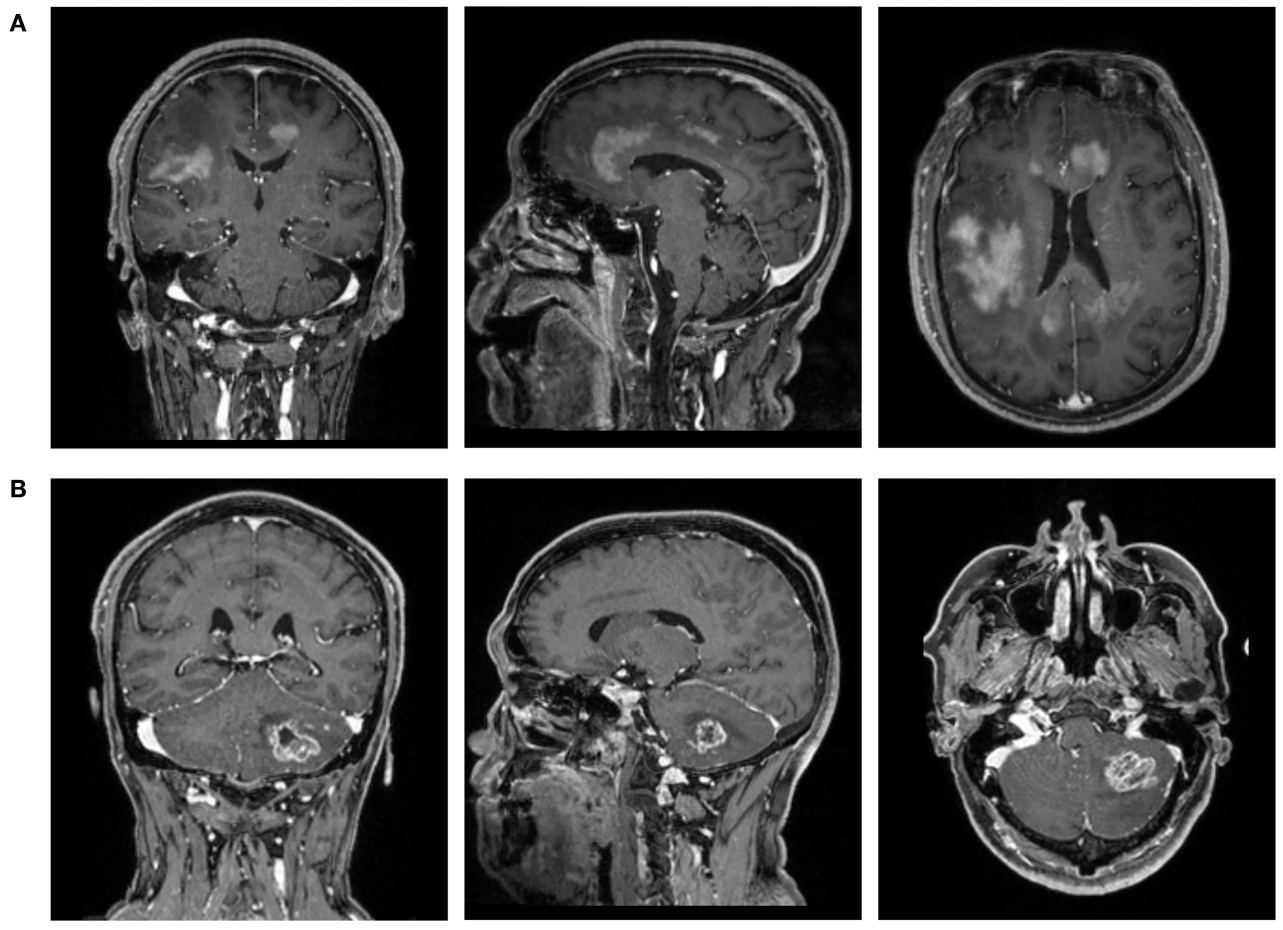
The magnetic resonance images (T1C) of patients with (A) primary central nervous system lymphoma (PCNSL) or (B) glioblastoma (GBM).
